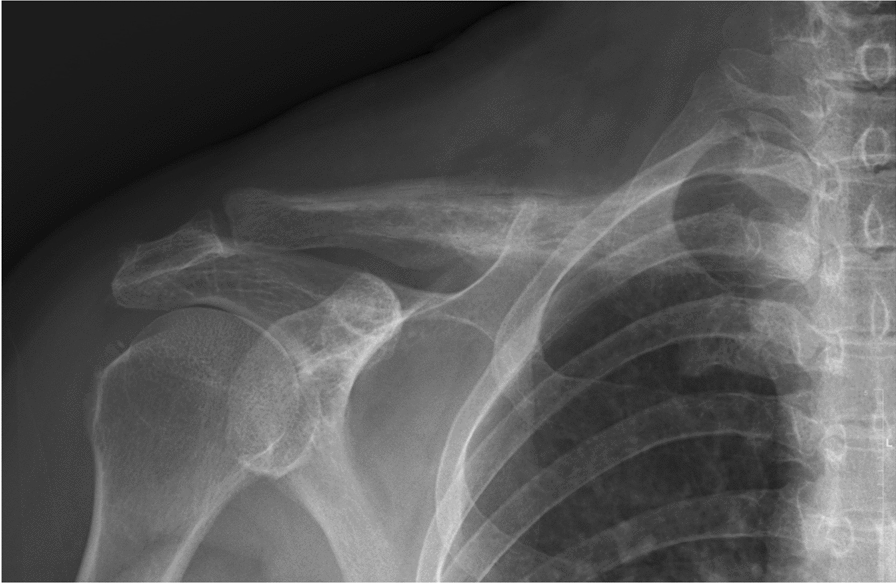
Plain radiograph of the right clavicle showed osteolytic moth-eaten appearance with osteosclerotic periosteal reaction
